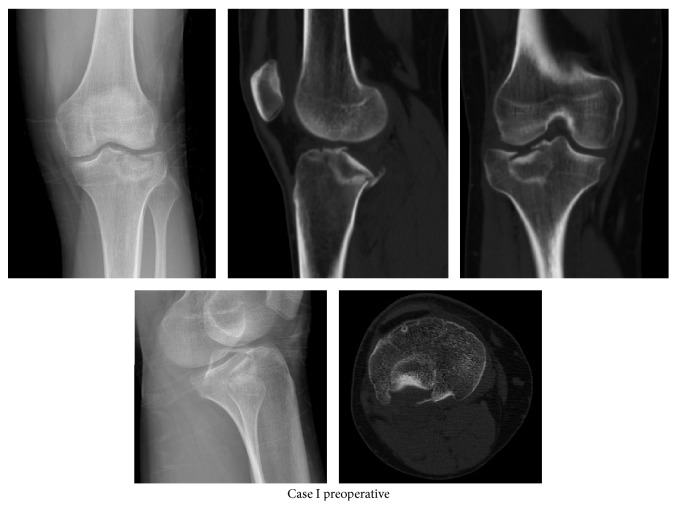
Preoperative radiographies and computed tomography images of Case I.
